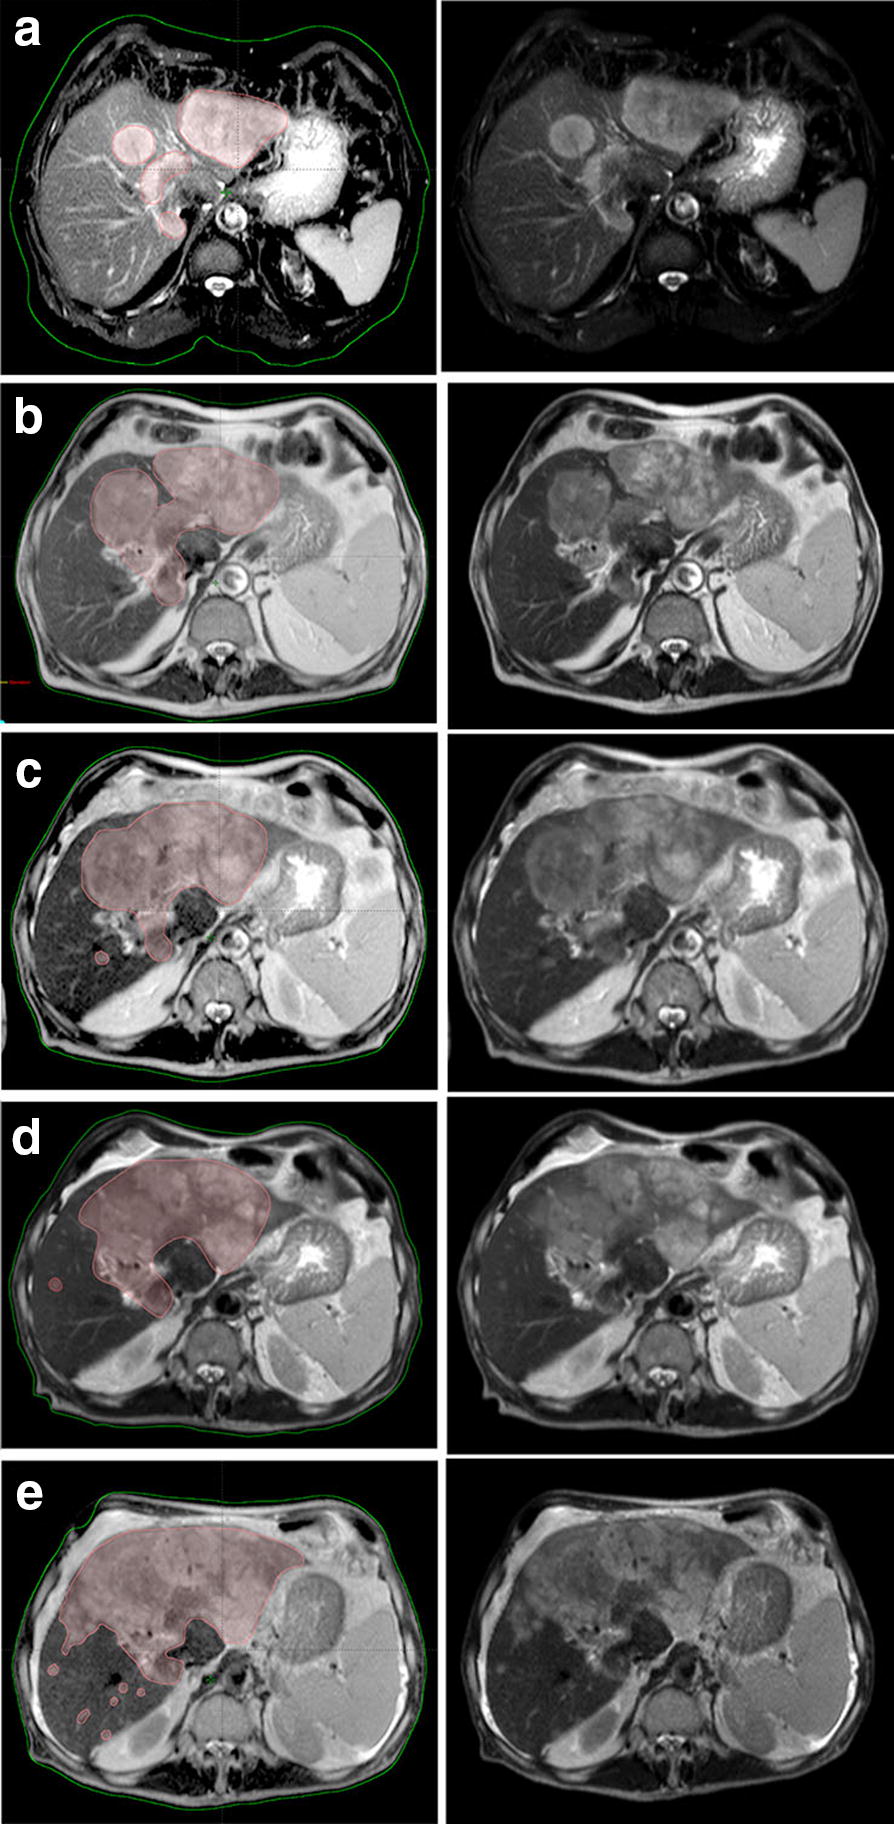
MRI tumor evaluation during treatment. Tumor area is highlighted on the left panel. Raw images are provided on the right panel. a Tumor evaluation at the stage of the initial CCA diagnosis (October 2015, was done with liver vein contrasting (7.5 ml Gadovist). Sum of diameters of target lesions equals 221 mm; b tumor progression after the best clinical practice care (July 2016). Sum of diameters of target lesions equals 278 mm (26% increase); c tumor growth during Sorafenib treatment (October 2016). Sum of diameters of target lesions equals 314 mm (16% increase); d tumor growth after Sorafenib treatment prior to start of Pazopanib (January 2017). Sum of diameters of target lesions equals 392 mm (35.3% increase). e Tumor progression after Pazopanib treatment (July 2017). Sum of diameters of target lesions equals 471 mm (35.7% increase if counting a as a reference or 20% increase if counting d as a reference)
